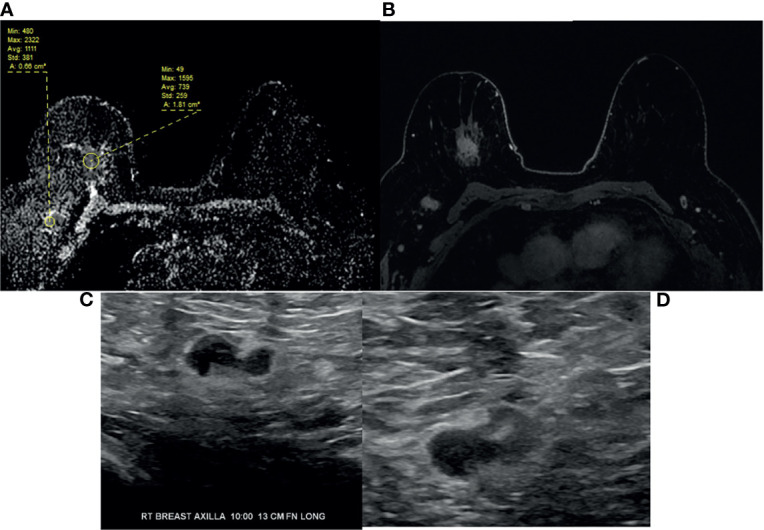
64-year-old woman with right breast 12:00 axis triple negative invasive ductal carcinoma and right axillary lymphadenopathy as seen on (A) ADC, (B) T1-weighted fat saturated post-contrast image. (C) Targeted ultrasound shows borderline cortical thickening of the right axillary lymph node. (D) Ultrasound-guided fine needle aspiration confirmed metastatic adenopathy. Note the difference in ADC values between primary breast and right axillary adenopathy, e.g., mean ADC 0.739 versus 1.111.
